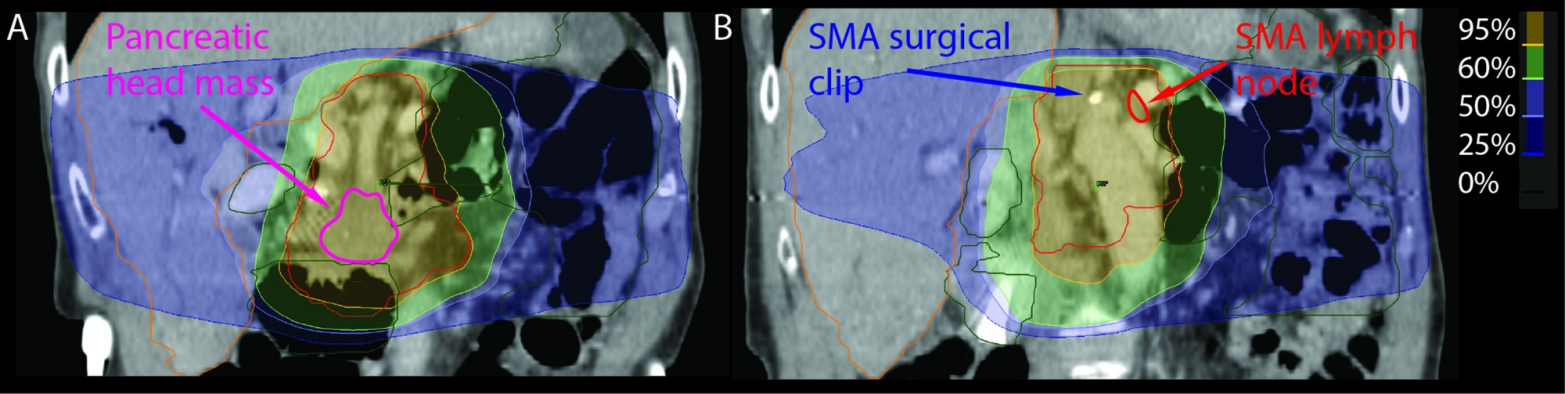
Coronal views of the isodose distribution for the original VMAT plan. The pancreatic head mass (A) is located anteriorly and inferiorly to the SMA node (B). A metal clip from a previous surgery, superior to the take-off of the SMA, served as a fiducial for the SMA node on CBCT. SMA: Superior mesenteric artery; VMAT: Volumetric modulated arc therapy; CBCT: Cone-beam computed tomography.
